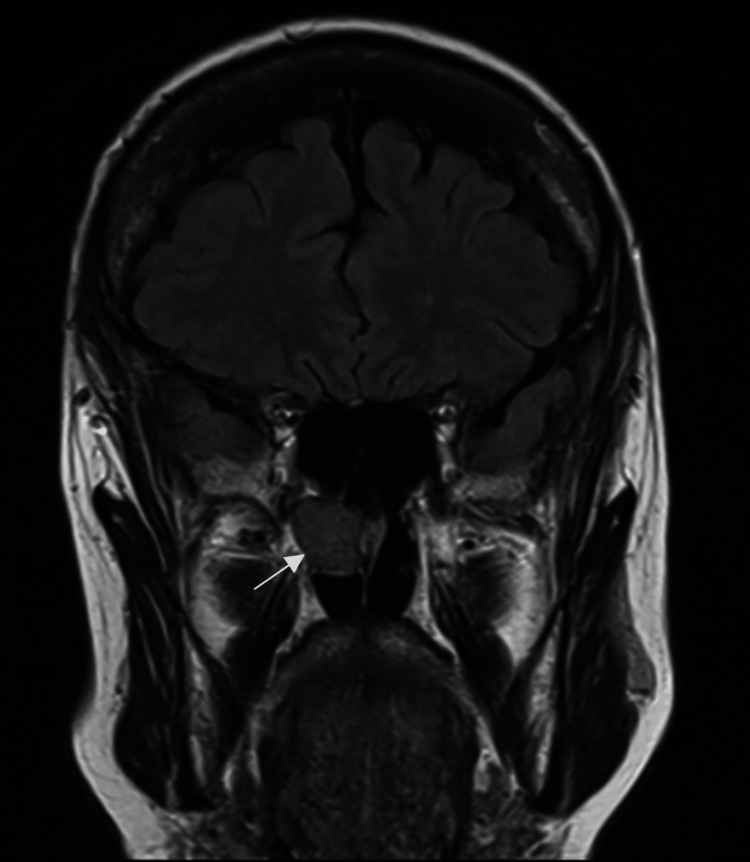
T1-weighted brain MRI without contrast. A hypointense lesion measuring approximately 16 mm, located along the posterior margin of the right nasal cavity.
